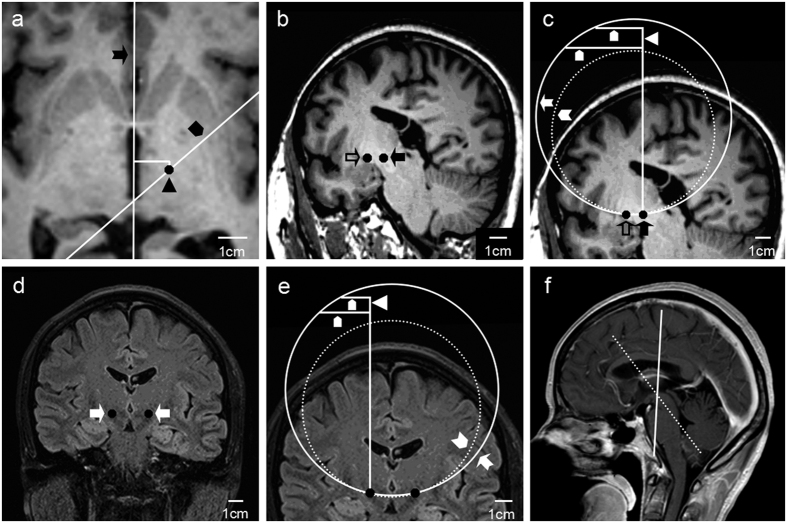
Design the “curved lead pathway” on MR images. (a–c) The “curved lead pathways” passing through the right STN and right GPi. a. The axial image of the AC-PC plane. The lead pathway plane (black pentagon) passes through the point (12, −3, 0) (black triangle) and forms a 49.4 degree angle with the medium sagittal plan (black dovetail arrow). (b) Reconstruct the lead path plane on the MRI-workstation of the device (Siemens Verio 3.0 T, Siemens AG, Erlangen, Germany). The lead pathway plane is orthogonal to the AC-PC plane, and passes through the right GPi (hollow arrow) and STN (black arrow). (c) Identify the “curved lead pathway” on the “lead pathway plane”. When r = 5 cm, “curved lead pathway” is indicated by the white dovetail arrow head. When r = 6 cm, the “curved lead pathway” is indicated by the white dovetail arrow, and the estimated locations of the positioning base and poles are indicated by the white triangle and white pentagon, respectively. (d–f) The “curved lead pathways” passing through the bilateral STN. (d) The lead pathway plane, which is orthogonal to the sagittal plane and perpendicular to the AC-PC plane, passes through the bilateral STN (white arrow). (e) Identify the “curved lead pathway” on the “lead pathway plane”. When r = 5 cm, the “lead pathway” is indicated by the white dovetail arrow head. When r = 6, “curved lead pathway” is indicated by the white dovetail arrow, and the estimated location of the positioning base and poles are indicated by the white triangle and white pentagon, respectively. The lead pathway passes through the parietal lobe. (f) Adjust the safe entry point of the lead by adjusting the location of the positioning frame. When the plane of the bottom frame (the XOY plane) is parallel to the AC-PC plane, the “lead pathway plane” is indicated by the solid white line, and the lead entry point is located at the parietal bone. If the bottom frame is leaned forward for 30–40°, the approximate position of the “lead pathway plane” is indicated by the dash line, and the lead entry point is located at the frontal bone.
